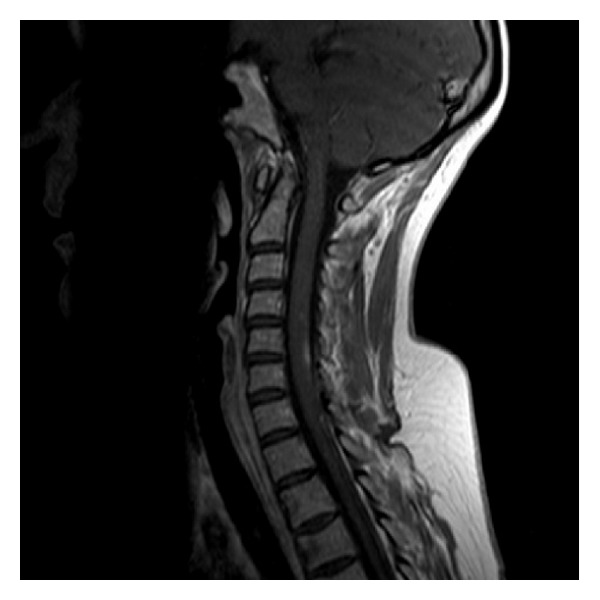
MRI spine showed a long intramedullary inflammatory lesion in the cord from C3 to the upper boarder of T1 on T1-weighted MRI imaging.
